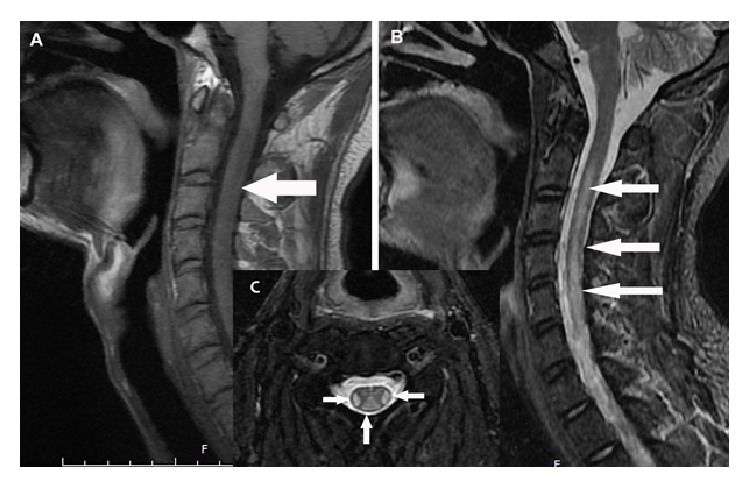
(A) T1-weighted MRI scans showing the dorsal spinal cord with hypointensity involving the posterior and lateral columns before treatment. (B) T2-weighted MRI scans showing the dorsal spinal cord with hyperintensity involving the posterior and lateral columns before treatment. (C) Transverse T2-weighted MRI scan of the cervical spinal cord at the C3 level demonstrating bilateral symmetric signal intensity within the dorsal and lateral columns (inverted V sign) before treatment.
